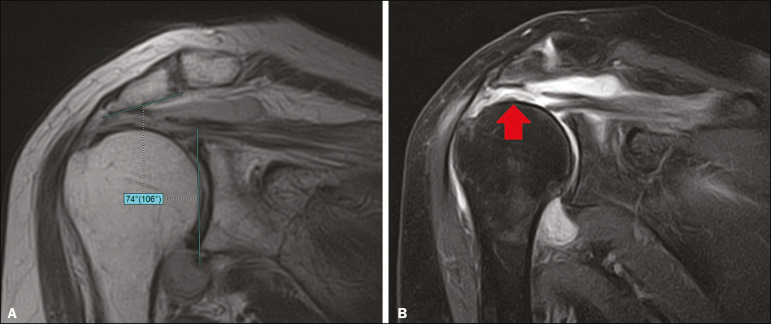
A 82 year-old man with signs of complete rupture of the supraspinatus tendon. A: Proton density-weighted image in the oblique coronal plane at the level of the acromioclavicular joint presenting a glenoacromial angle of 74°. B: T2-weighted image with fat saturation in the oblique coronal plane at the level of the supraspinatus tendon showing an interruption of the tendon fibers (arrow), characterizing complete rupture.
